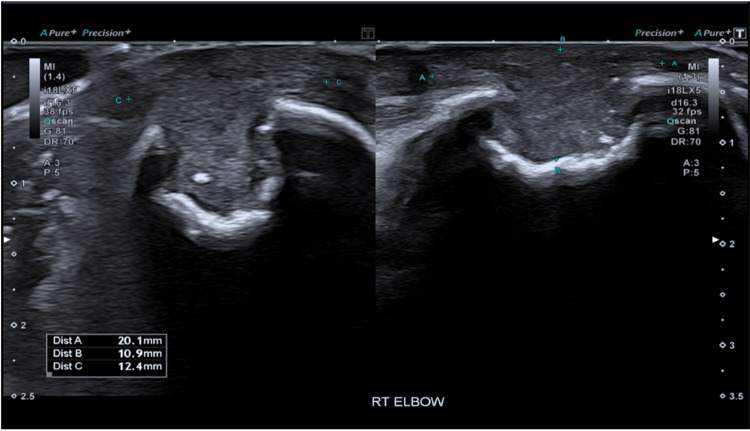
Ultrasound Right Elbow: The superficial component of the previously described olecranon bursa is essentially collapsed. The component eroding into the olecranon is still evident. There is no surrounding hyperaemia to suggest active inflammatory changes.
